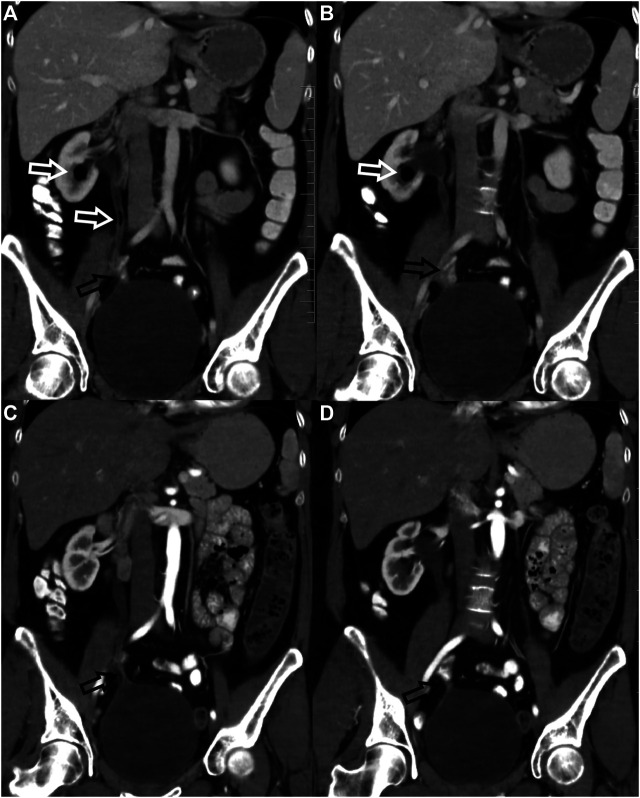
Clinically-silent uretero-enteral fistula. Uretero-enteral fistula was found incidentally 7 years after RT in a patient after right ureter-to-bladder reimplantation due to early postoperative stenosis. The patient did not suffer from any clinical manifestations, urinalysis was normal. CT revealed air bubbles in the right ureter and renal calyx (white arrows–(A,B) due to the uretero-enteral fistula located in the vicinity of ureter reimplantation. There was no evidence of patent fistula on CT 1 year later (C,D), the site of ureter reimplantation was clearly visualized (arrows).
